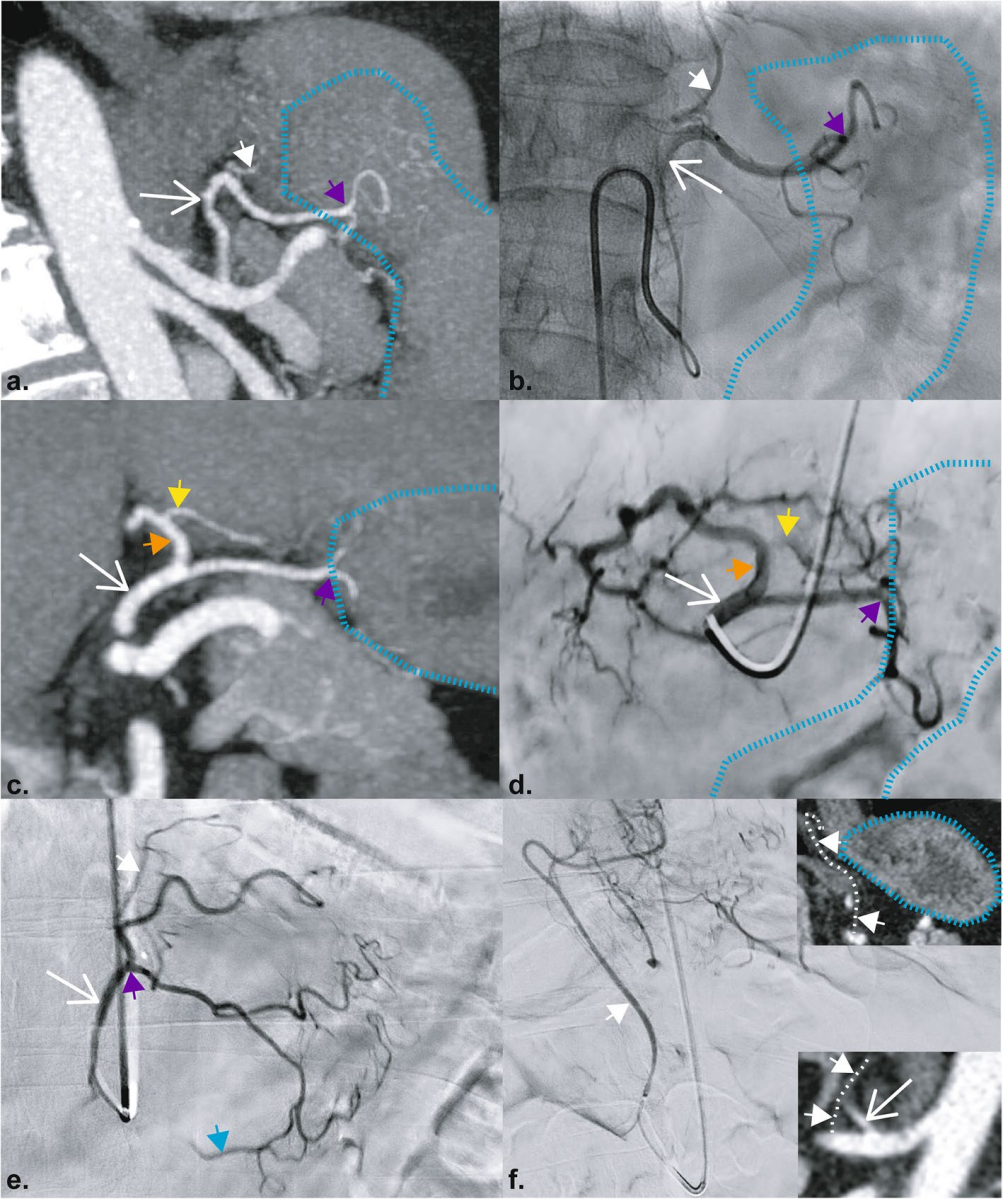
Examples of variant LGA anatomy on CT and catheter angiography. a CT angiography of 1b LGA classification. b Corresponding catheter angiogram of the same participant. c CT angiography of 3e classification of the LGA. There is an accessory left hepatic artery (orange arrow) with an accessory LGA (yellow arrow). d corresponding catheter angiogram in the same participant. e Catheter angiography of a participant with 2c classification with the GOJ artery arising from the superior branch of the LGA and a large right gastric artery anastomosis with flow reversal (blue arrow). f Catheter angiogram of the GOJ artery originating directly from the coeliac trunk. The top insert shows the course of the GOJ artery (white dashed line) in the coronal plane on and the bottom insert shows its origin just distal to the LGA from the coeliac trunk in the sagittal plane. Key: LGA, long white arrow; GOJ artery, short white arrow and white dashed line; accessory LGA, yellow arrow; the bifurcation of the LGA, purple arrow; accessory left hepatic artery, orange arrow; right gastric artery anastomosis, blue arrow; stomach outline, blue dashed line
